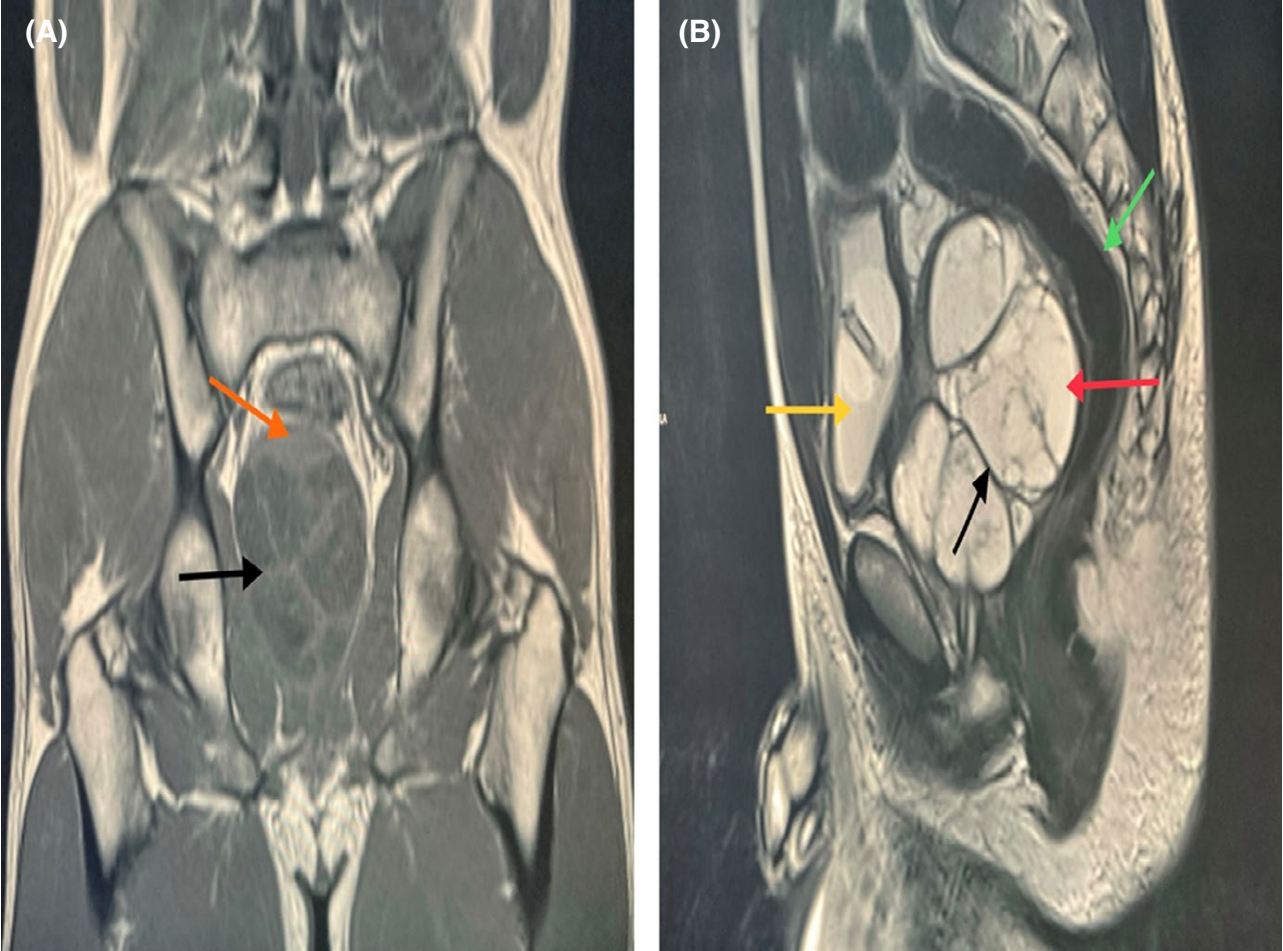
(A) Preoperative post‐contrast coronal MRI T1, showing cystic lesion with enhancing septa (black arrow) and enhancing soft tissue component (orange arrow). (B) Preoperative MRI Sagittal T2, showing large cystic lesion (red arrow) with septations (black arrow) exerting mass effect on rectum (green arrow) and urinary bladder (yellow arrow) which appeared under filled with Foley's catheter in situ
